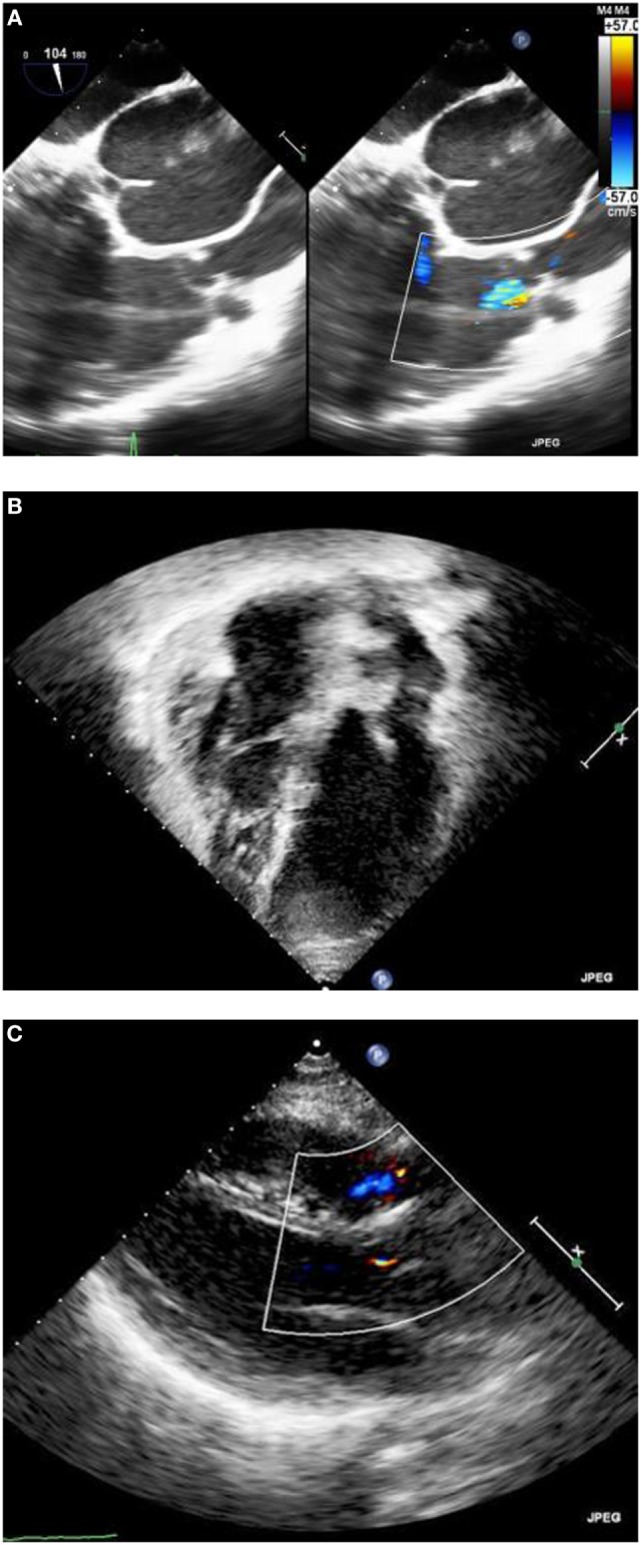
(A) Intra-operative transesophageal echocardiography after repair, showing trivial residual degree of pulmonary valve regurgitation. (B) Pre-discharge trans-thoracic echocardiography with the four chamber views. (C) Pre-discharge trans-thoracic echocardiography with the long-axis view.
